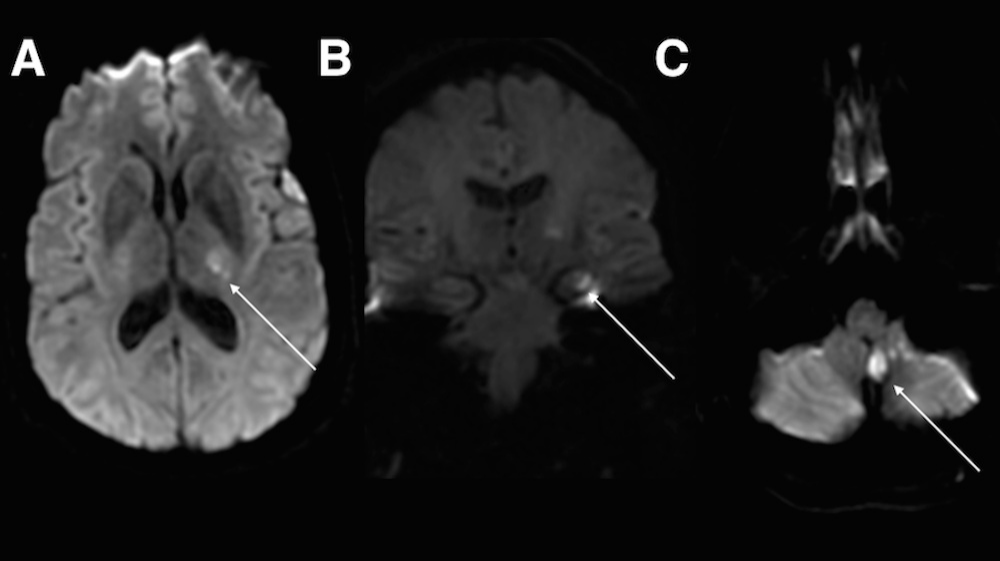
Magnetic resonance imaging (MRI) demonstrating acute infarct. Axial diffusion-weighted imaging (DWI) showing acute infarct in the left lateral thalamus (arrow - A), coronal DWI showing acute infarct in the left hippocampal tail (arrow - B) and axial DWI showing acute infarct in the left cerebellar tonsil (arrow - C).
